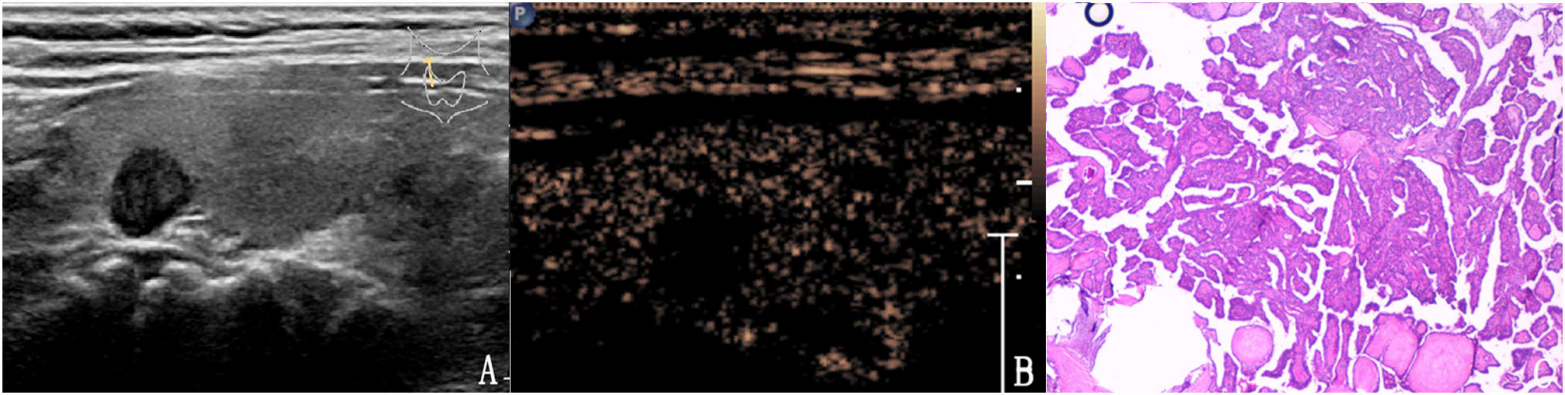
The case of a 47-year-old man with a 7.8 × 6.8 × 7.5 mm solid mark hypo-echoic nodule in the right lobe of the thyroid. (A) Conventional two-dimensional image showing that the nodule had two malignant indicators (solid, mark hypoechoic) and was classified with a TI-RADS score of 4a. (B) Ultrasound contrast image showing low enhancement. The modified version of the TI-RADS combined with CEUS returned a score of 4b and the diagnosis was a malignant nodule. (C) Pathological image of the lesion, a PTC.
